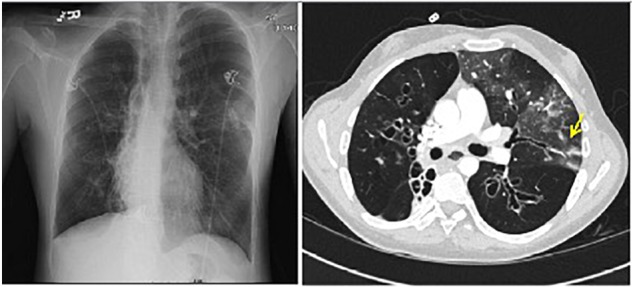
Left: chest radiograph demonstrating cystic bronchiectasis. Right: CT image of chest showing a left upper lobe infiltrate on a background of cystic bronchiectasis (yellow arrow).
